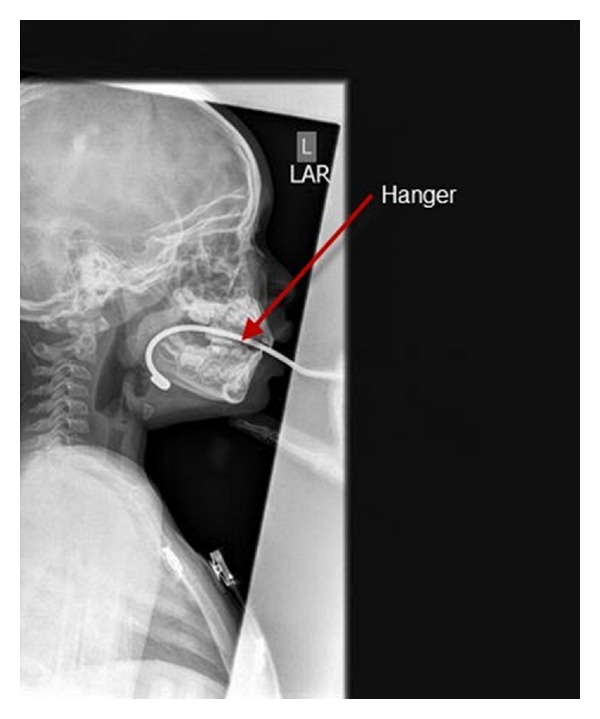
Lateral neck X-ray showing foreign body.
